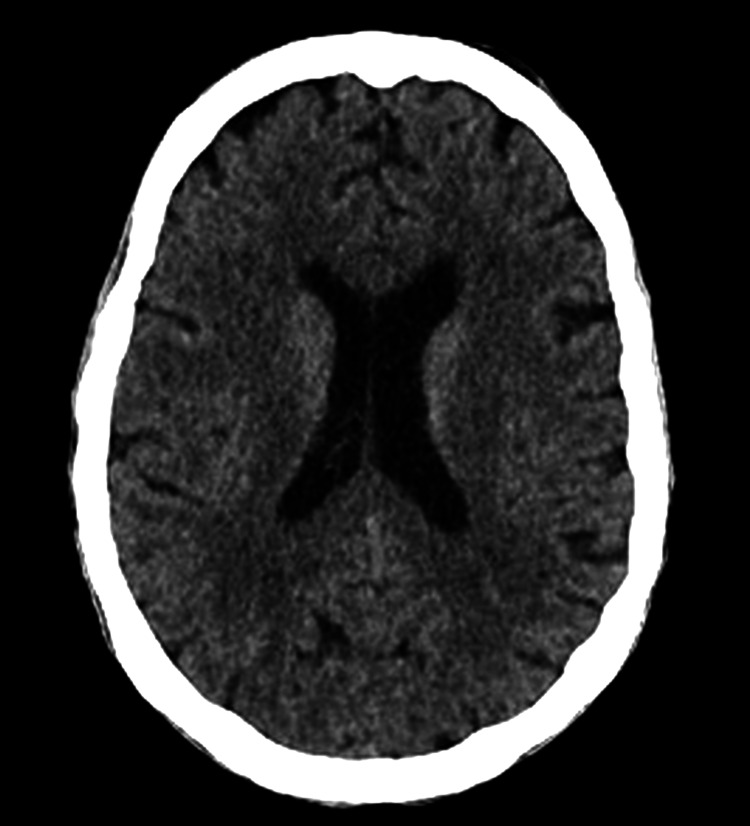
Non-contrast CT of the brain. Non-contrast axial CT of the brain demonstrating preserved gray–white matter differentiation and symmetric ventricular system, with no evidence of acute intracranial hemorrhage, mass effect, or ischemic lesions. These findings highlight the limited sensitivity of structural neuroimaging in the early stages of autoimmune encephalitis, despite significant neuropsychiatric manifestations.
